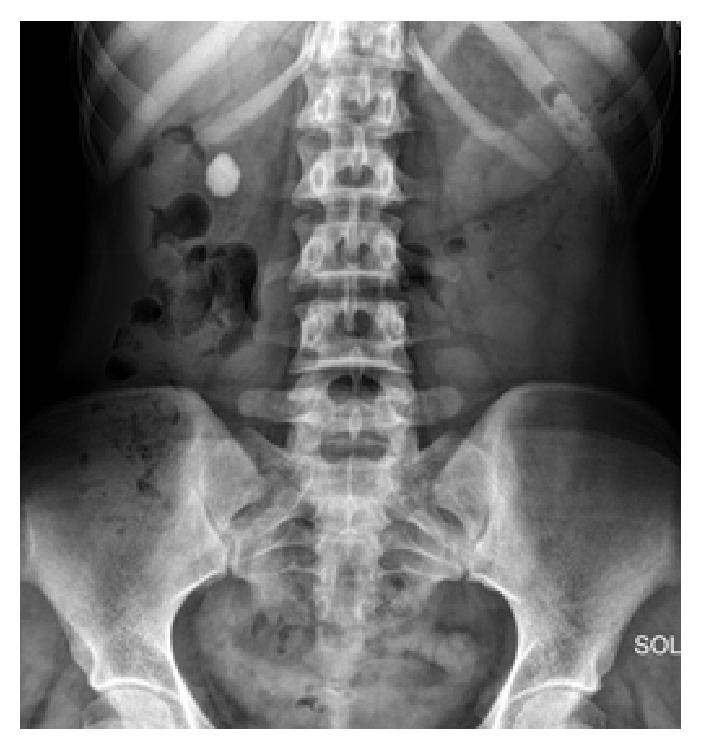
Preoperative kidney, ureter, and bladder X-ray of patient.
